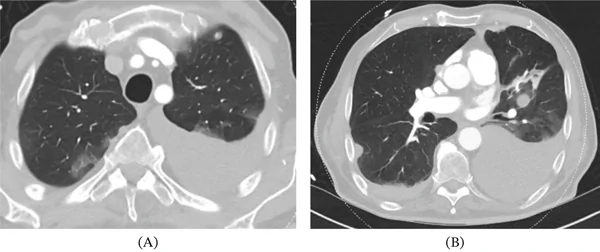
Initial chest computed tomography (CT), lung window. (A) Ground‐glass opacities in the upper lobes bilaterally, with a left‐sided pleural effusion. (B) Multiple pleural‐based nodules along the costal and mediastinal pleura in the right hemithorax and within the interlobar fissure of the left lung. A smaller right‐sided pleural effusion is also present.
